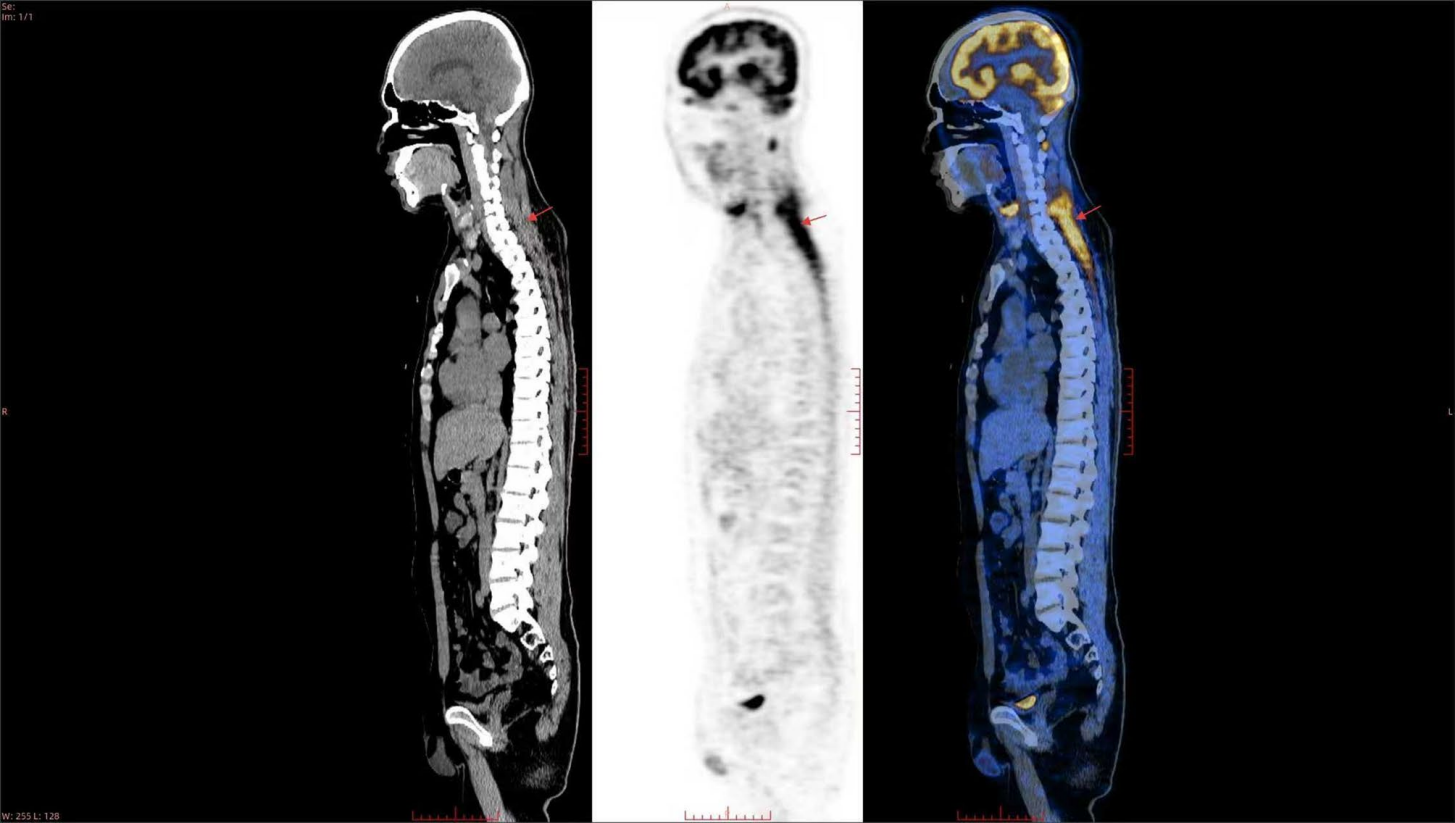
Case 1; ^18^F-FDG PET/CT. Diffuse metabolic activity was identified in the bilateral neck muscles (arrows)
